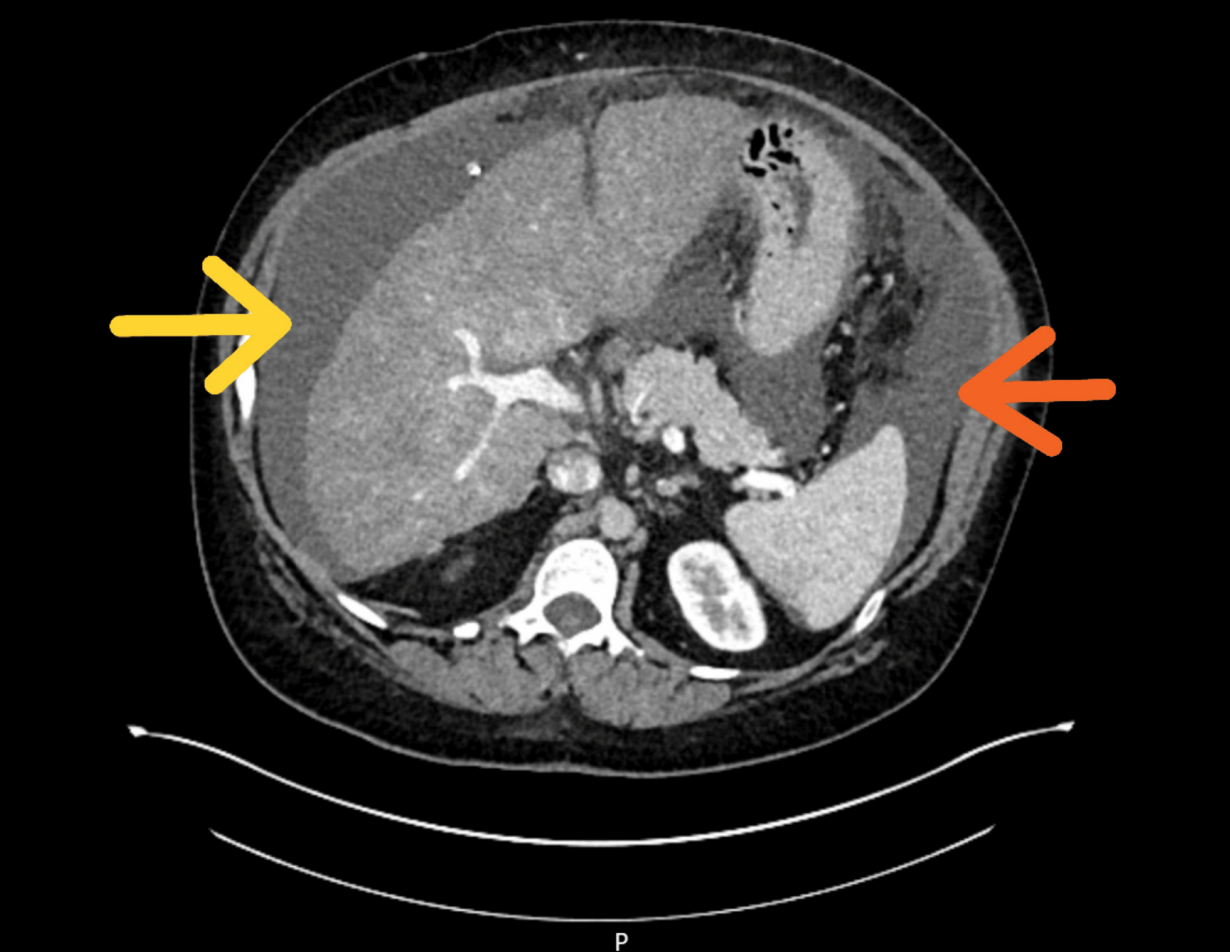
CT scan of the abdomen and pelvis showing ascites and small volumes of fluid in the perihepatic (yellow arrow) and perisplenic areas (orange arrow)
